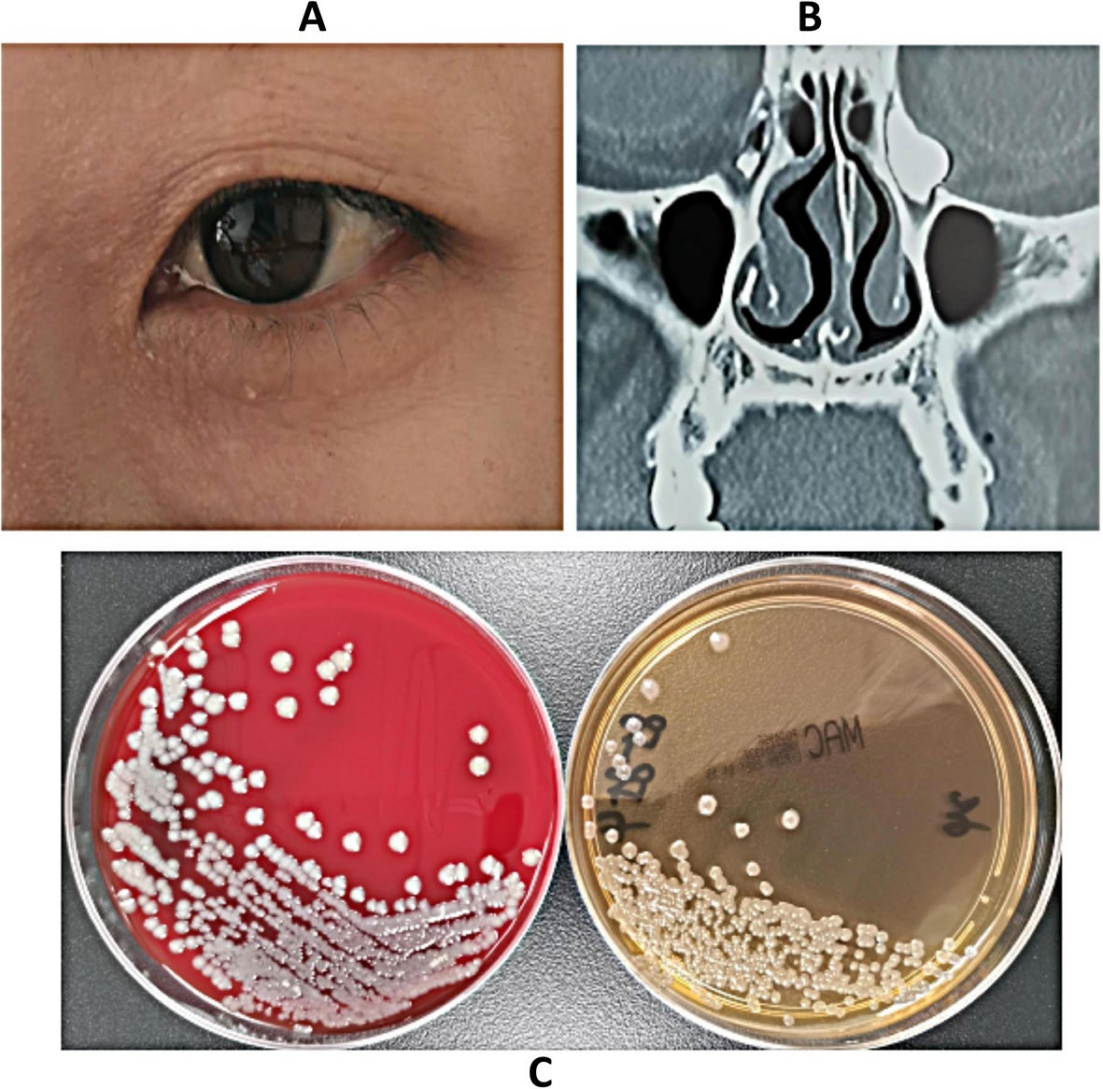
A 31-year-old female patient presented with left dacryocystitis that did not show any improvement for 6 months despite treatment with 0.488% levofloxacin ophthalmic solution. CT imaging revealed the presence of a lacrimal sac cyst. After 24 h, *Stenotrophomonas maltophilia* was cultured from the secretion of puncta lacrimalia
